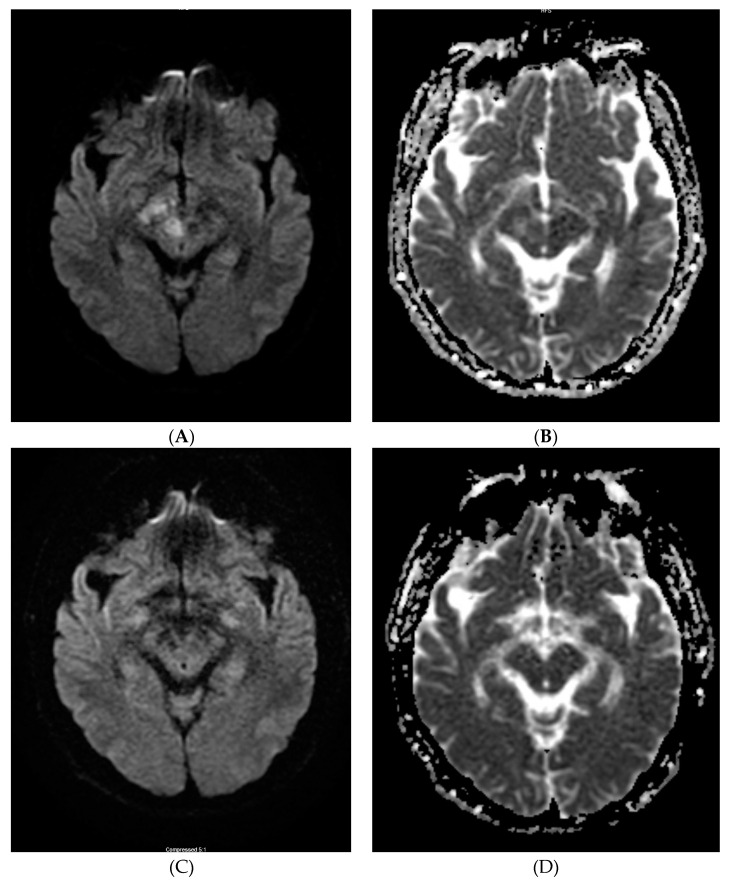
Selected MRI diffusion-weighted imaging (DWI)/apparent diffusion coefficient (ADC) imaging from initial (A,B from Jan 2017) and last (C,D from May 2017) MRI.
